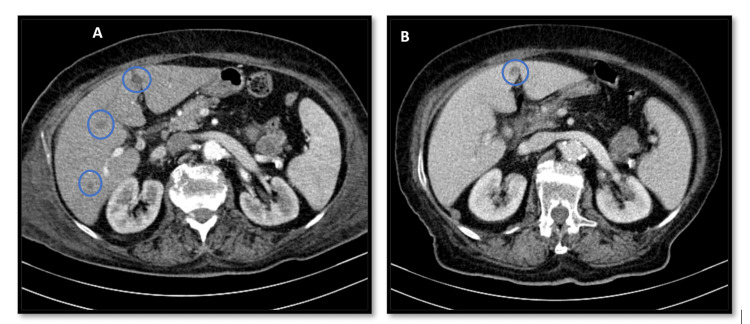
First (A) and second (B) computed tomography scans. (A) Three lesions in the liver and (B) only one is seen.
